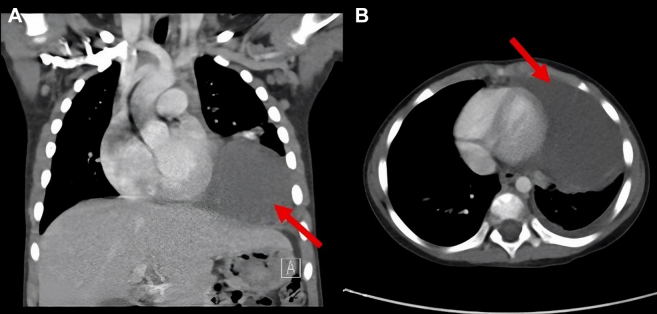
CT Chest With Contrast Computed tomography chest with contrast performed on day 3 of admission, with coronal plane (A) and axial plane (B), demonstrating a large 9.4 × 6.2 × 5.4 cm fluid density in the left cardiophrenic recess, believed to represent a pericardial cyst (red arrows).
